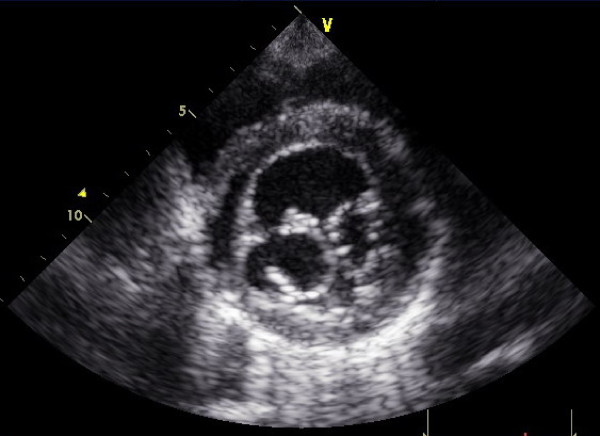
Parasternal short-axis view shows double-orifice mitral valve by two-dimensional echocardiography.
